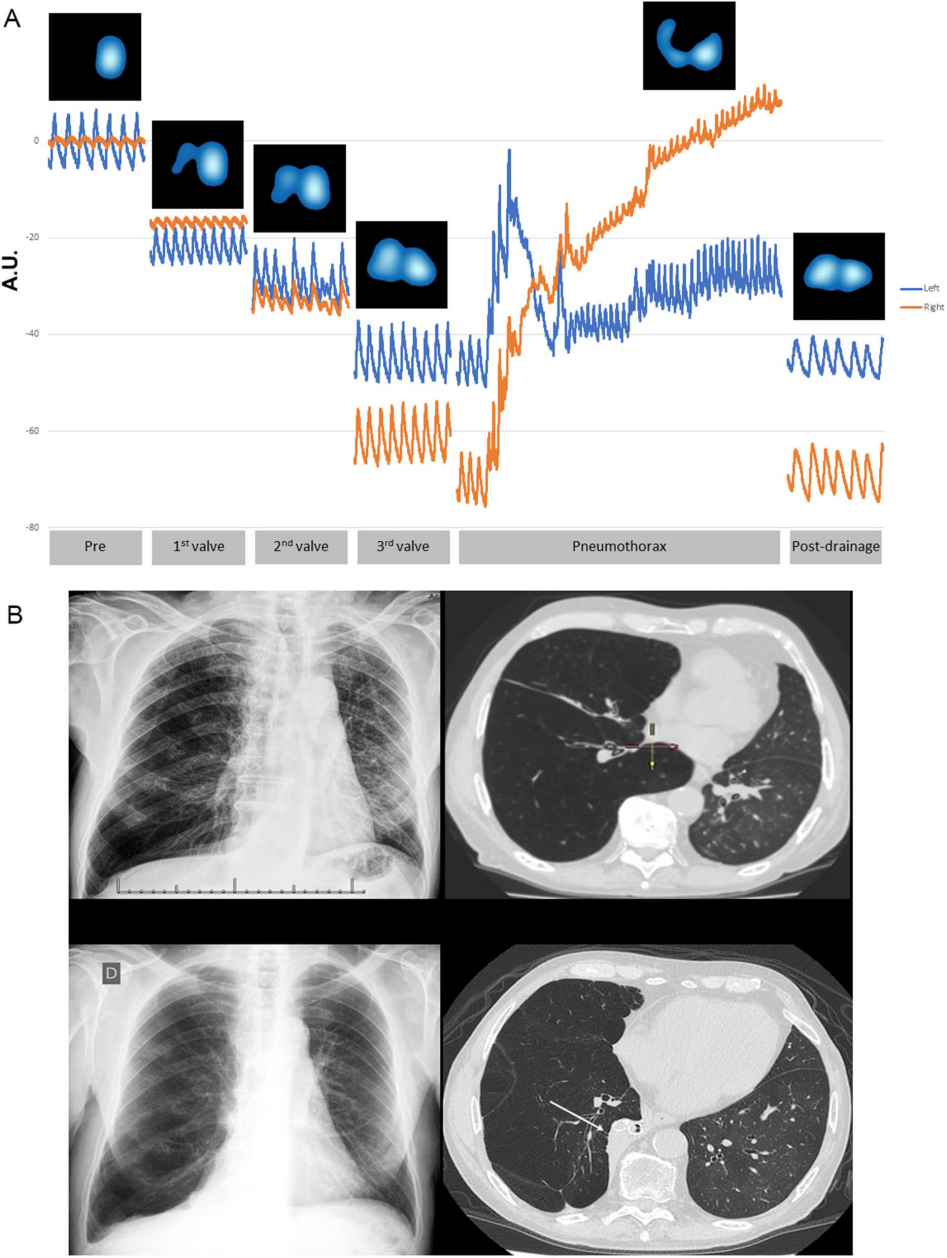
Observations from the second patient. A 71-year-old male with emphysema underwent a left single lung transplant seven years before. He developed progressive disabling dyspnea starting five years after transplantation, mainly because of progressive hyperinflation of the native lung. The forced expiratory volume in one second (FEV1) dropped from 1860 mL to 580 mL, and the post-transplant Modified Medical Research Council (mMRC) dyspnea scale shifted from 1 to 3 within the last year when he became full-time oxygen-dependent because of bronchiolitis obliterans and severe hyperinflation of the native lung. The observations from this patient focused on the periods before (briefly after sedation: Pre), during, and after bronchoscopic lung volume reduction (BLVR) with one-way endobronchial valves (EBV)-Zephyr^TM^ (See Supplementary Video S1, Additional File 5). Electrical impedance tomography (EIT) continuous real-time imaging showed the changes in regional ventilation, and changes in regional end-expiratory lung impedance (EELI). The EIT images were sub-segmented into two regions-of-interest (ROIs): right (orange) and left (blue) hemithorax. A Chartis^TM^ catheter was advanced into the right lower lobe bronchus, and a negative collateral ventilation (CV-) pattern was recorded by the Chartis^TM^ console. Three EBV Zephyr^TM^ valves were placed in the right lower lobe segmental bronchi. Upon completion of the BLVR procedure following the withdrawal of the laryngeal mask during recovery from the sedation, with the patient still in the operating room, he had a sudden coughing spell followed immediately by dyspnea, right-sided chest pain, and a steep decrease in peripheral capillary oxygen saturation (SpO2), from 98 to 80%. Immediately before the sudden coughing spell (See Supplementary Video S1, Additional File 5), the EIT tracings showed a quick rise in the regional EELI of the right hemithorax (orange EIT tracing), combined with a corresponding significant attenuation of the regional ventilation within the same right ROI (A), which are the typical changes of a pneumothorax in the EIT signals [23]. As the patient?s respiratory condition rapidly deteriorated, becoming critical, in addition to the accompanying real-time EIT tracings and images for pneumothorax altogether, allowed us to proceed with an emergency right-sided chest tube drainage. It was followed by improved dyspnea and pain, yielding stabilization of the ventilatory condition and SpO2 within a few minutes. (A) shows the EIT tracings and images of the events before (briefly after sedation: Pre), during each valve placement, and throughout the pneumothorax event, including post-drainage (See Supplementary Video S1, Additional File 5). The patient recovered in the operating room, and the air leak from the chest tube resolved gradually until its removal on the fifth day after the drainage procedure. Three months after the BLVR procedure, SpO2 increased from 89.6 to 93%, the FEV1 and the forced vital capacity (FVC) showed an increase of 130 mL and 250 mL, respectively. The dyspnea scale dropped from mMRC 3 to 2.(B): Chest radiograph and computed tomography scan of this patient showed in the upper panel (before BLVR) the hyperinflated right lung, primarily due to the right lower lobe displacing the mediastinum towards the left side. In the lower panel (three months after BLVR), the right lower lobe atelectasis with the endobronchial valves in place (arrow) and the mediastinal shifted towards the midline
